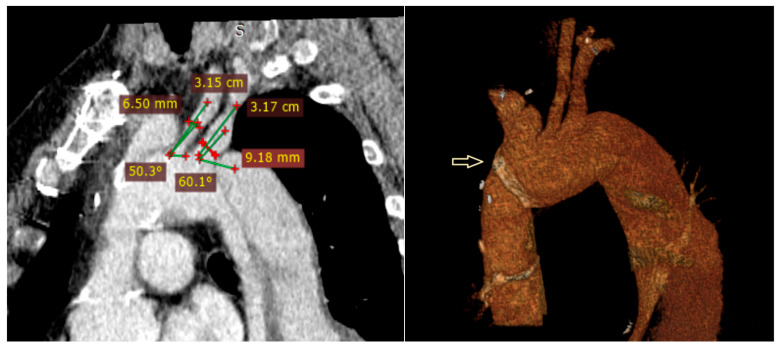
Preoperative CTA coronal section measurements of the diameter of the left carotid and subclavian arteries and the angle formed with the arch, three-dimensional images of the aortic arch, and the suture line from the previous operation (arrow).
